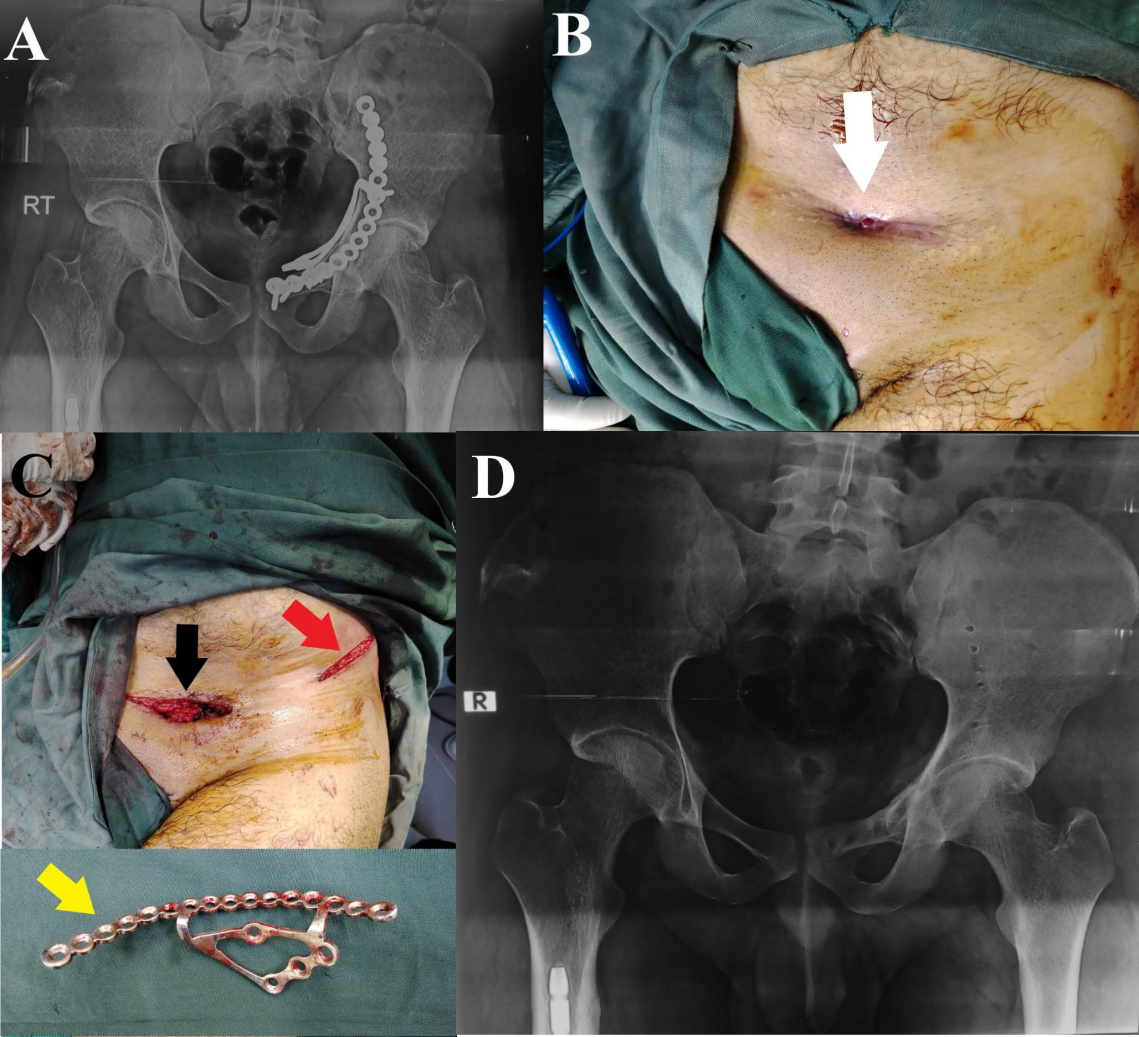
27-year-old male patient who had a T-type fracture treated by ORIF, hardware was performed through a modified Stoppa approach and a lateral window after 24 months of the index surgery. A, preoperative. B, a clinical image showing the discharging sinus (white arrowhead). C, an intraoperative image showing the modified Stoppa approach (black arrowhead), the ilioinguinal approach iliac window (red arrowhead), and the retrieved implant (yellow arrowhead). D, postoperative anteroposterior radiograph. (Patient No. 13 in Supplementary File 2)
